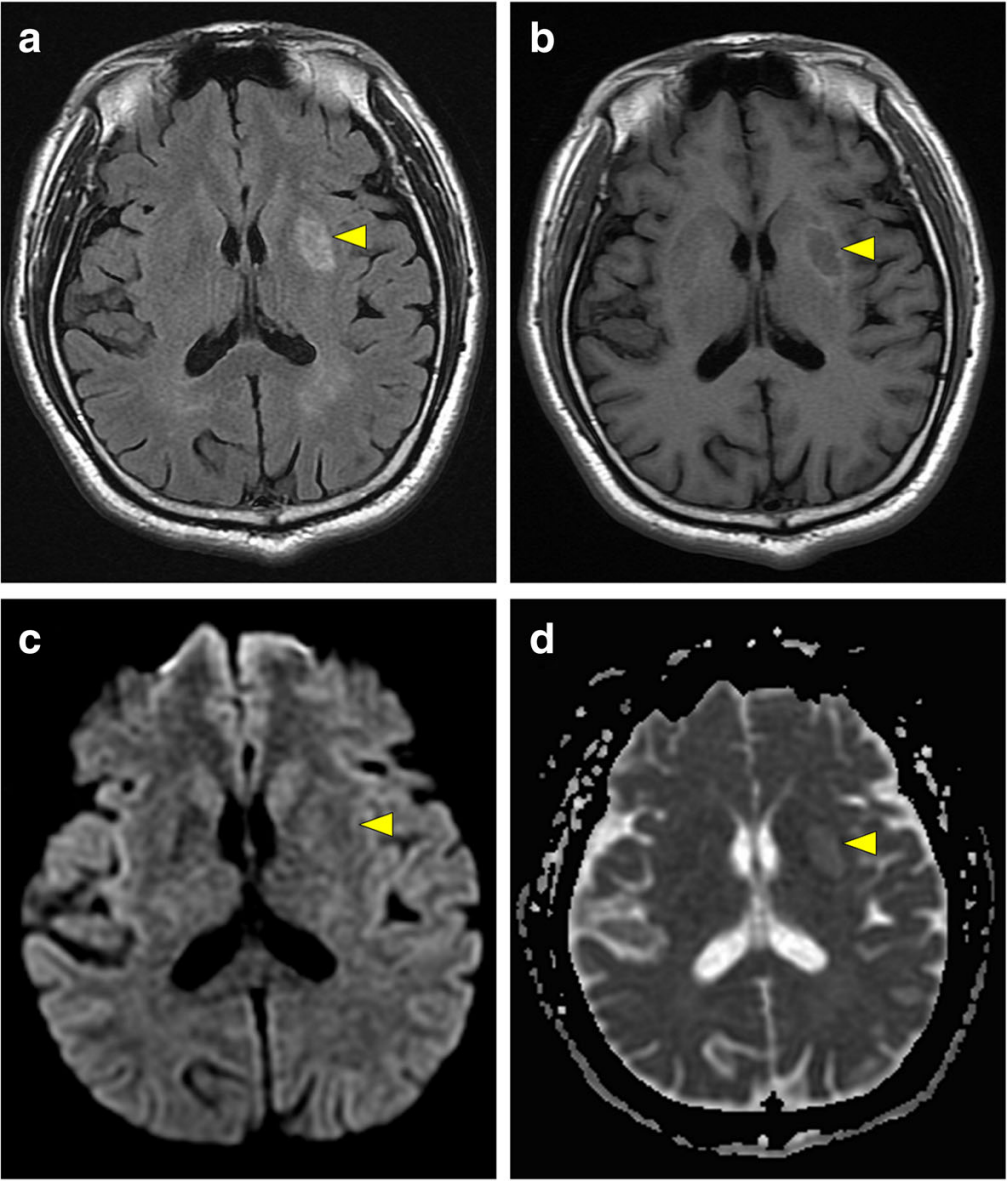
MR scans of the brain at the level of the striatum; FLAIR image (a), T1-weighted image (b), diffusion-weighted image (c), and apparent diffusion coefficient map (d), revealing the presence of a subacute to chronic infarct in the left putamen (arrowheads)
